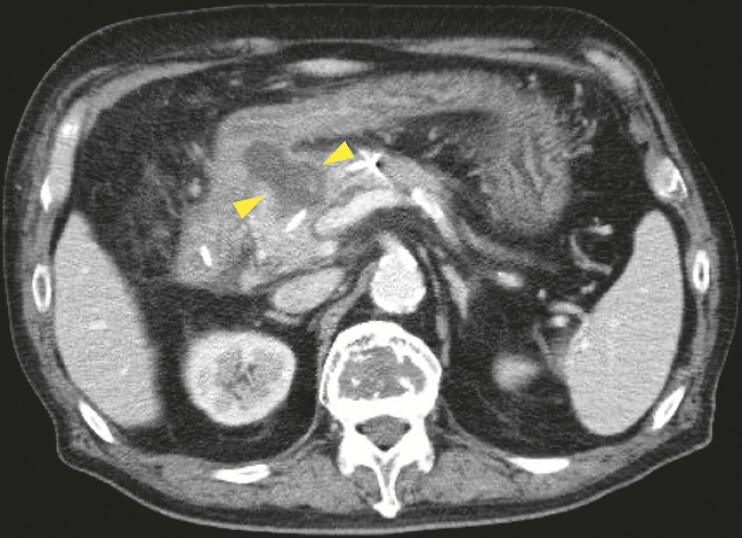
Follow-up CT demonstrating pseudocyst shrinkage (arrowhead). CT, computed tomography.
